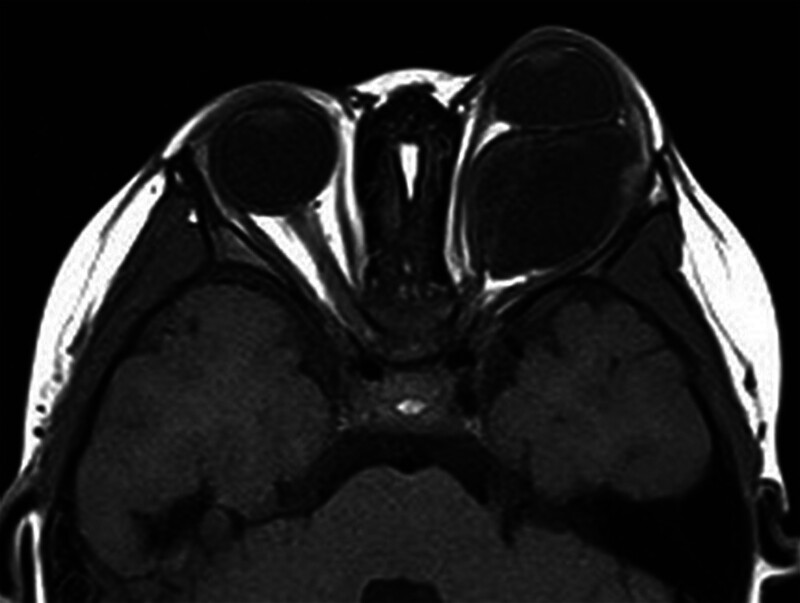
MRI depicting the lesion with hypointense signals on T1-weighted images (T_1_WI) and hyperintense signals on T2-weighted images (T_2_WI). MRI = magnetic resonance imaging, T_1_WI = T_1_-weighted images, T_2_WI = T2-weighted images.
